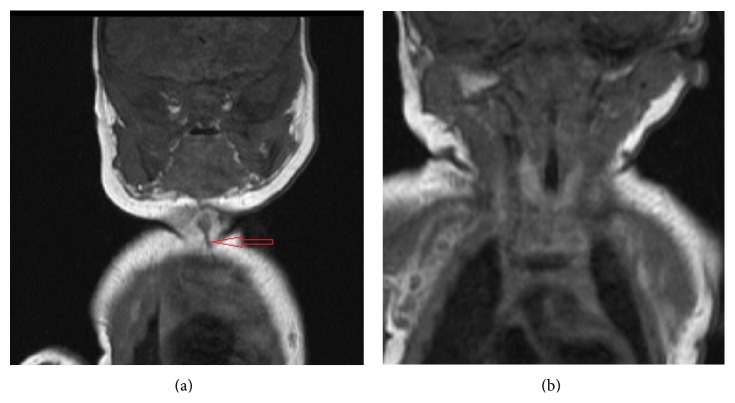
(a) Coronal T1 weighted MR image of neck demonstrates low T1 signal vertical midline cleft in cutaneous/subcutaneous region (open red arrow). (b) Coronal T1 image demonstrates normal right and left lobes of the thyroid.
